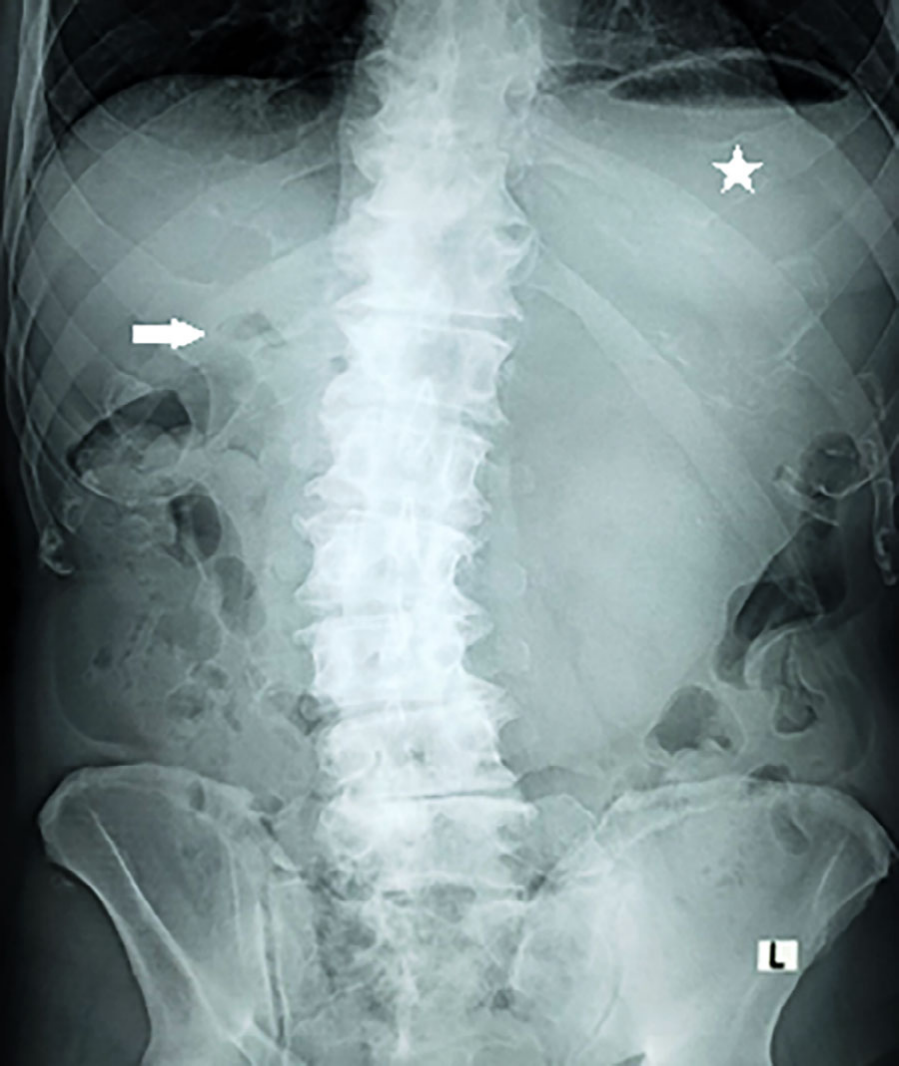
Plain abdominal radiography demonstrating pneumobilia (arrow) within the right upper quadrant and enlarged stomach (star).
